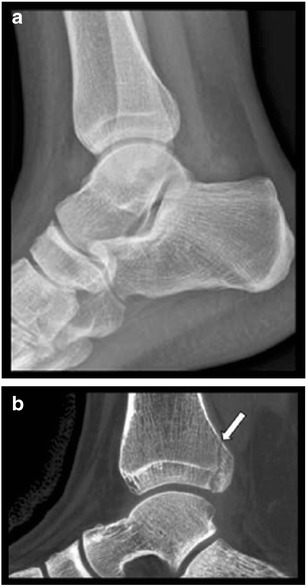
Fracture of the posterior malleolus in a 16-year-old female. a. CR (lateral view) of the ankle shows no evidence of fracture. b. Sagittal CBCT reconstruction showing a non-displaced malleolus tertius fracture (arrow). CBCT clearly demonstrates intra-articular extension of the fracture
